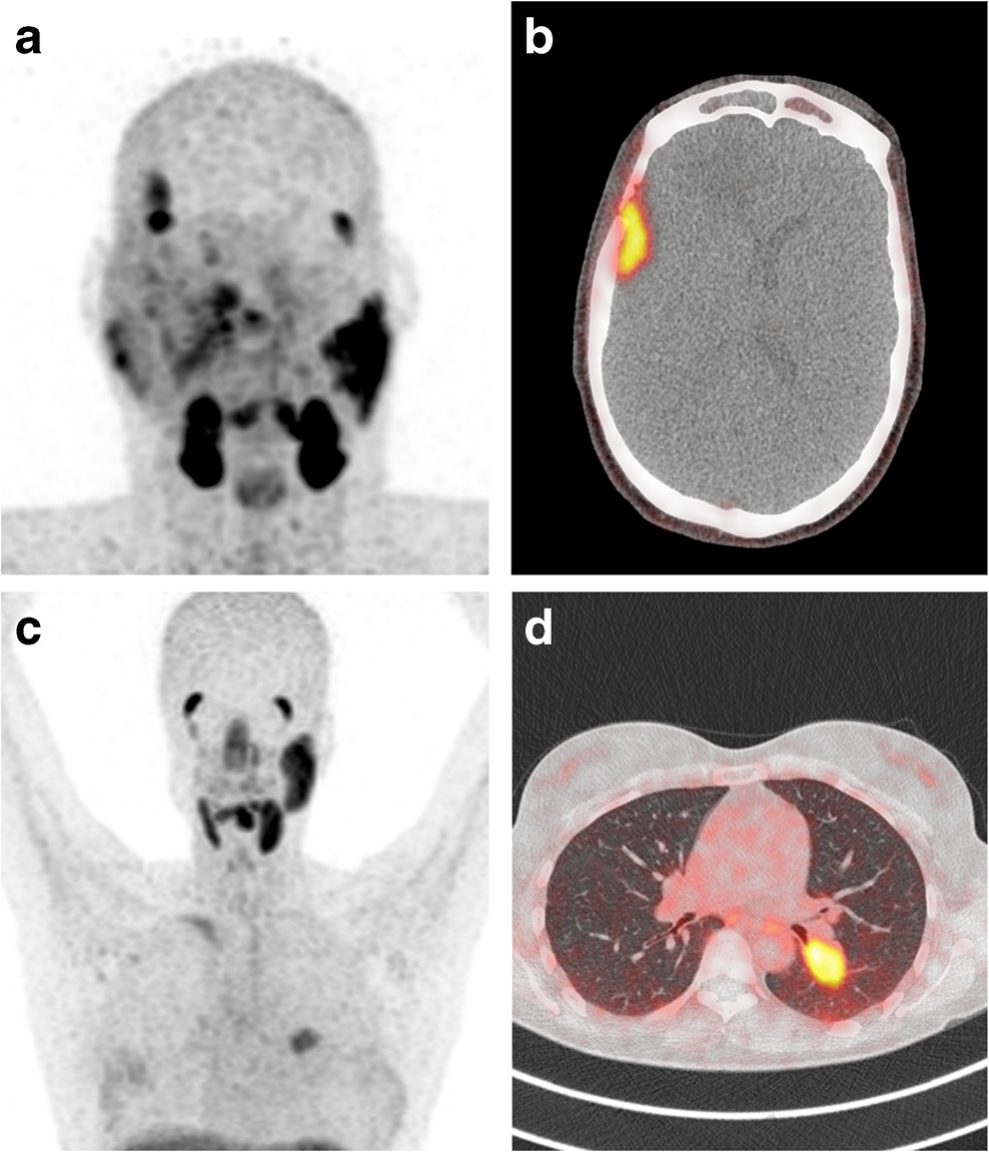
Overview of PSMA PET (a and c) and axial PSMA PET/CT slides (b and d) of PSMA-ligand uptake in AdCC. Patient no. 1 (a and b): nasopharyngeal recurrence and leptomeningeal metastasis; Patient no. 7 (c and d): local recurrence in the right maxillofacial region and pulmonary metastasis
